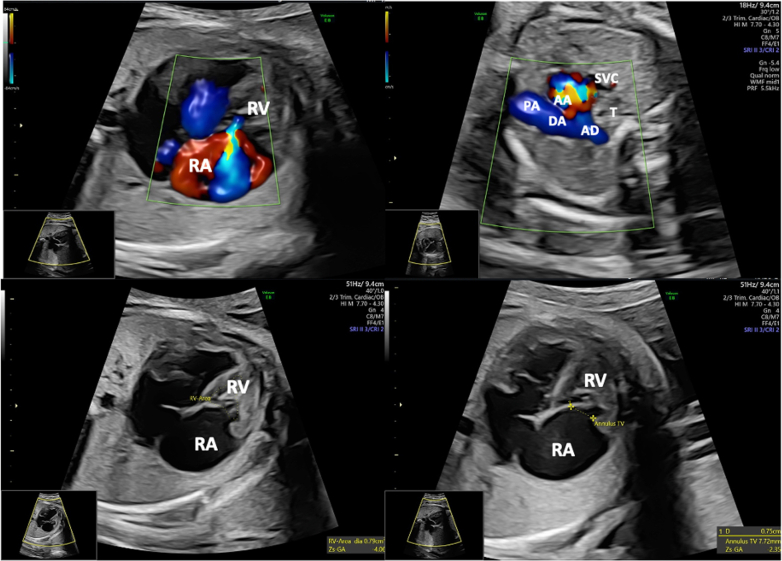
Fetal Echocardiographic Images at 32 Weeks of Gestation Showing Features of Tricuspid Valve Dysplasia and Pulmonary Valve Dysplasia With Intact Interventricular Septum (Top Left) Color Doppler 4-chamber imaging reveals severe tricuspid regurgitation with retrograde flow into the right atrium. (Top Right) Three-vessels and trachea view showing reversed blood flow from the aortic arch to the pulmonary artery. (Bottom Left) Measurement of the hypoplastic bipartite right ventricle area. (Bottom Right) Measurement of the tricuspid valve annulus, with a *z*-score of −2.41, indicating borderline right ventricular development. AA = aortic arch; AD = descending aorta; DA = ductus arteriosus; PA = pulmonary artery; RA = right atrium; RV = right ventricle; SVC = superior vena cava; T = trachea.
